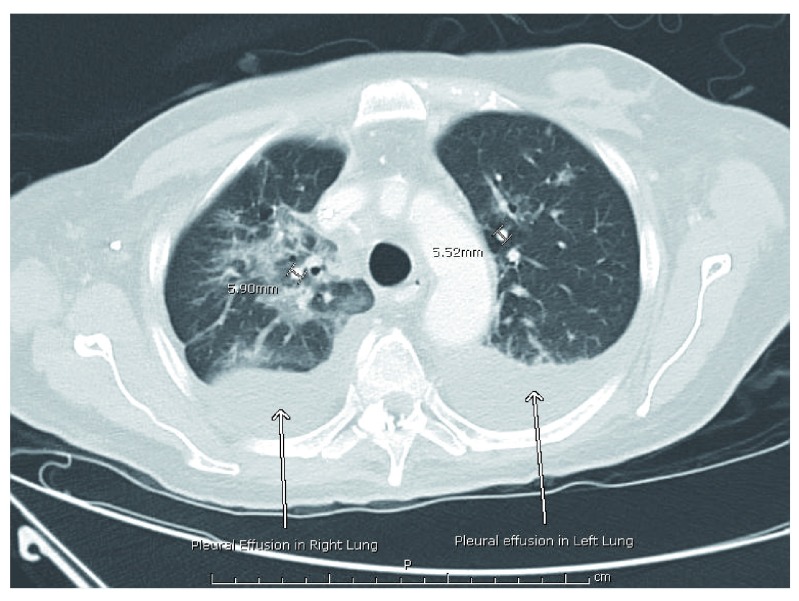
CT of the chest on admission to hospital. CT of the chest demonstrating several nodular opacities throughout both lungs. Two nodules are measured to show size. Arrows point to pleural effusions on both lungs.
